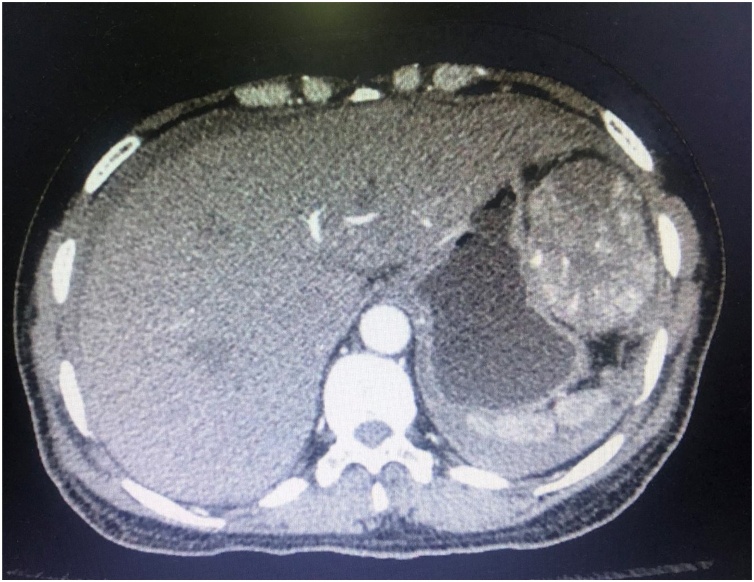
Contrast enhanced computed tomography demonstrated segment 2 and 3 liver lesion with wall defect and perihepatic hematoma.
